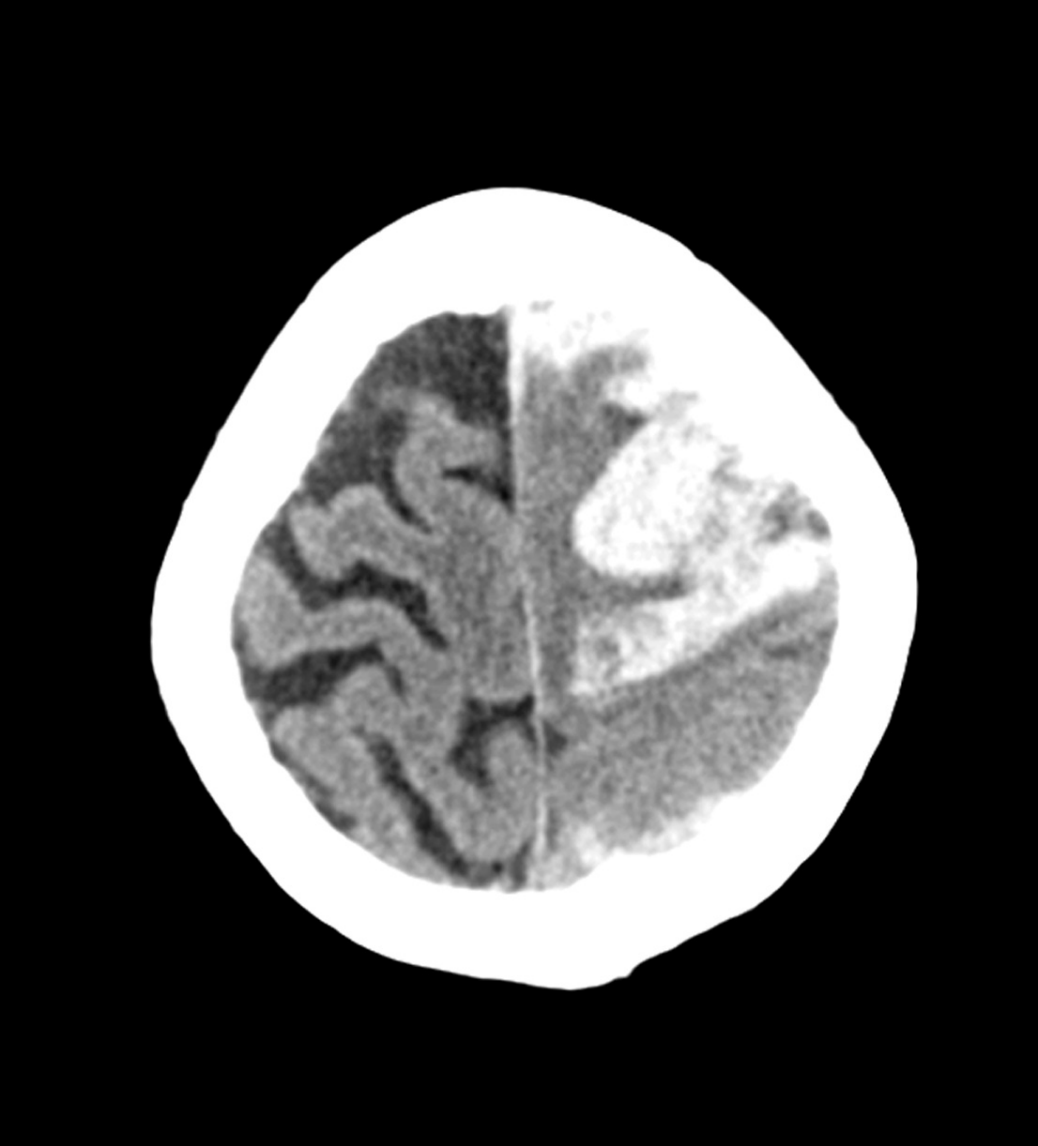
Axial non-contrast CT showing left frontoparietal hematoma
